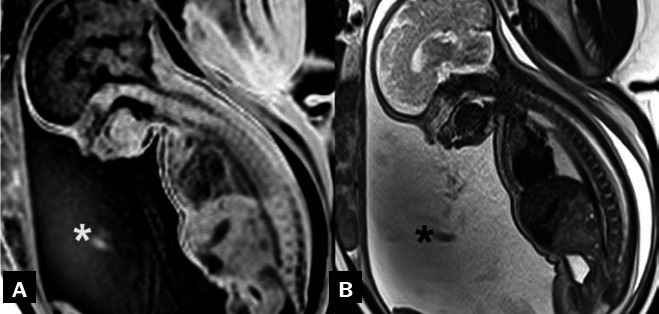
Fetal MRI. Sagittal T1- and T2- weighted sequences
